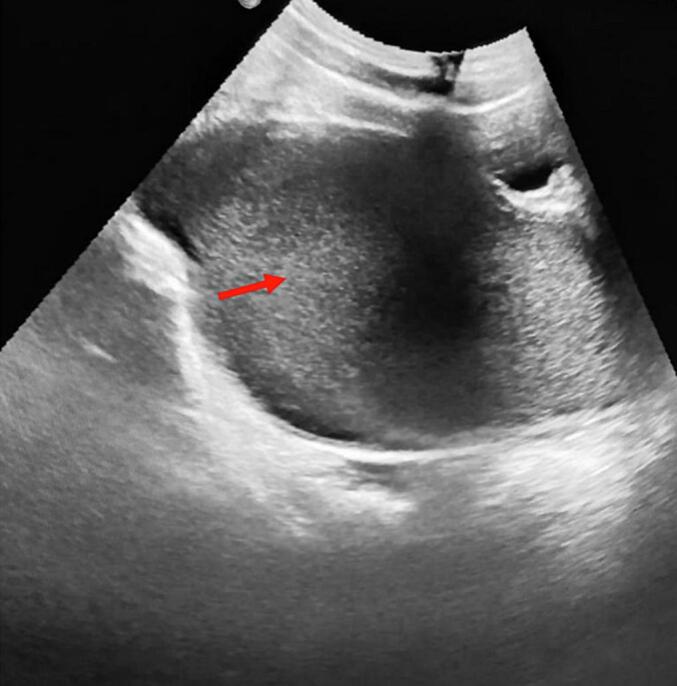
Surpubic ultrasound view of retro-vesical, median, hypoechoic fluid containing a fine echogenic spot (red arrow). (For interpretation of the references to colour in this figure legend, the reader is referred to the web version of this article.)
